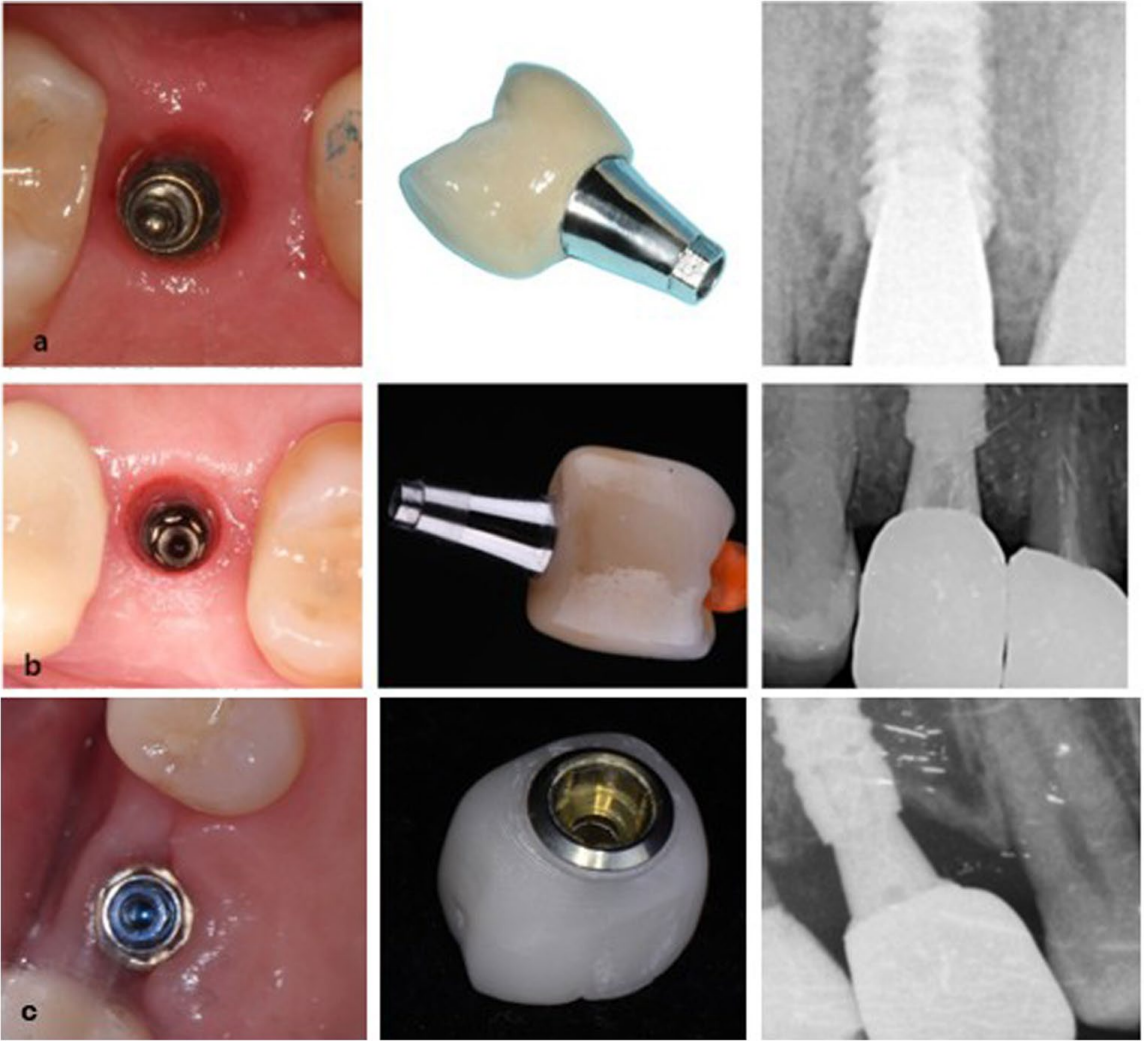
Different options for placing implant-supported restorations. From left to right: Occlusal view of the implant connection; Image of individual crown; Radiographic image: a Direct to implant. b Ti-Base. c Intermediate abutment
